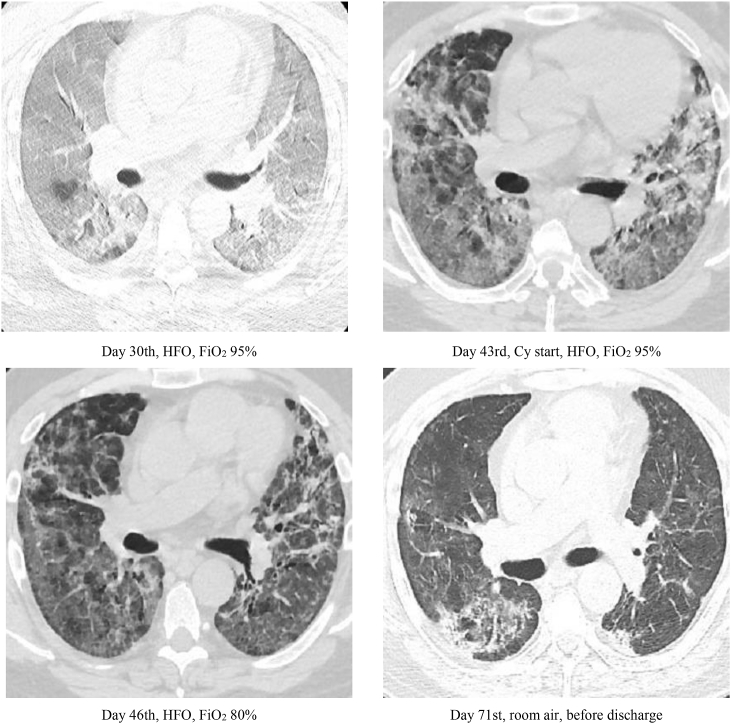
Lung CT scans of Patient 1 (days of the disease).
